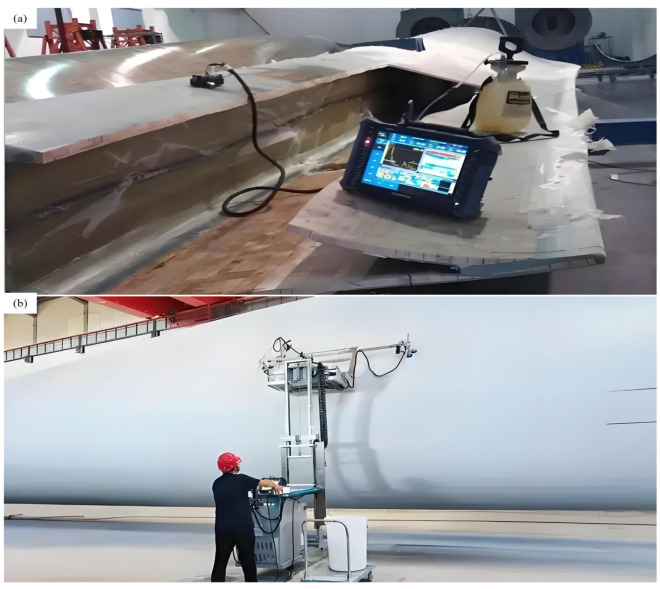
Ultrasonic phased array flaw detector. (a) Blade phased array detector. (b) Automatic scanner based on phased array. (Source: NISYO-GOYO).
